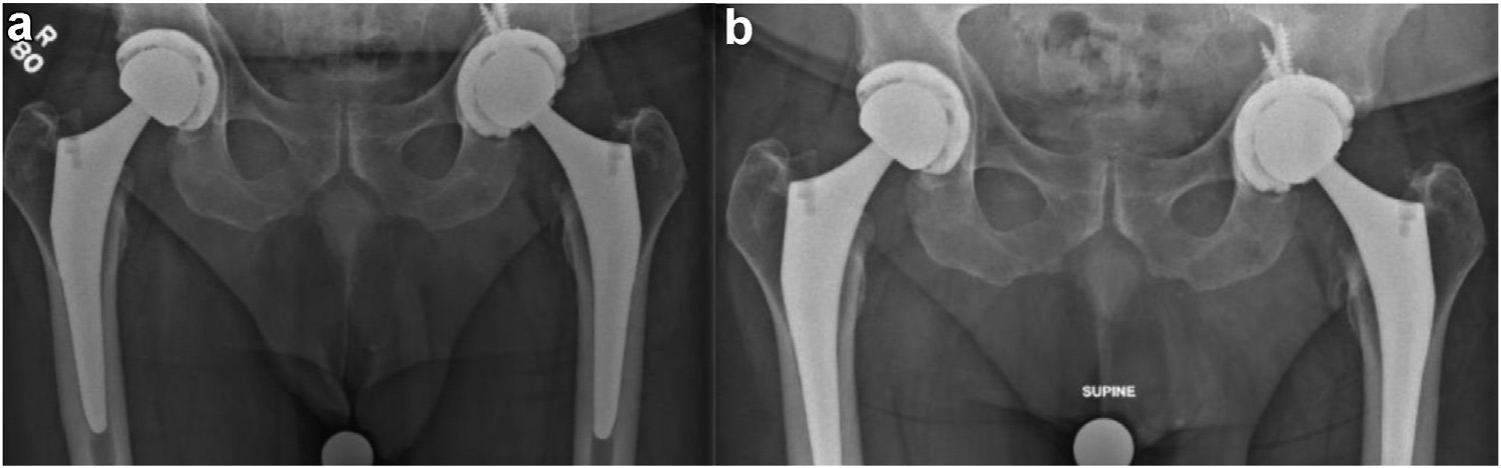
Postoperative left revision THA radiographs. Anteroposterior (AP) pelvis radiographs (a) 1 month and (b) 6 months postoperatively of left revision THA show well-aligned bilateral THA with no signs of complication.
